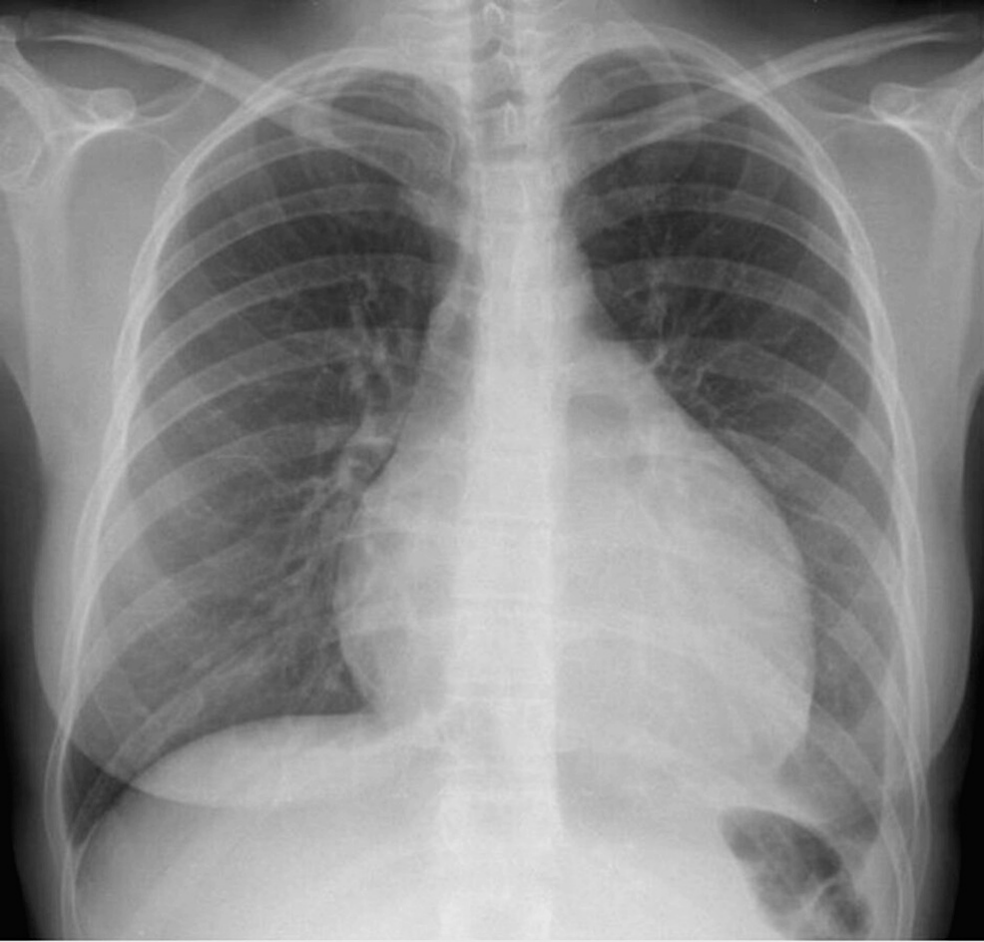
Chest radiography (posteroanterior view) at admission revealing enlarged cardiac silhouette and a small volume left pleural effusion
